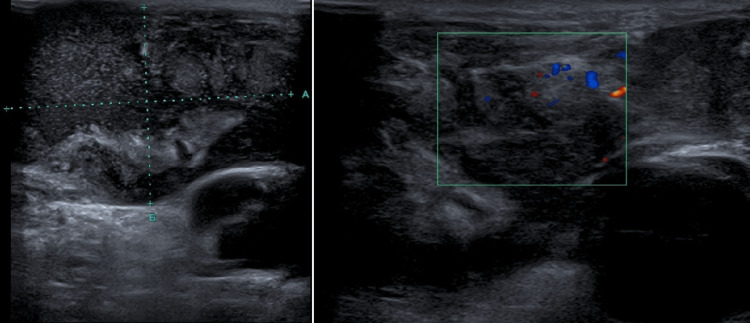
Ultrasound of the lesion showing a heterogenic structure with vascularization
